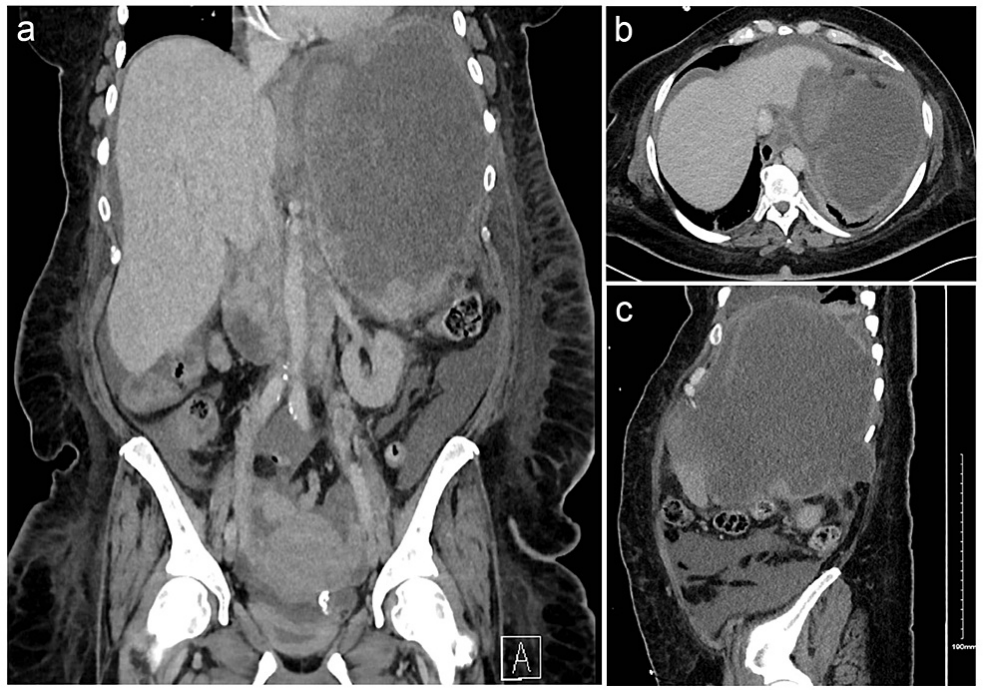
CT abdomen/pelvis with IV contrast, coronal (A), axial (B), and sagittal (C) views on re-admission demonstrating a large heterogeneous cystic septated retroperitoneal mass in the left upper quadrant causing significant mass effect on adjacent organs. The mass is poorly defined anteriorly with increased perisplenic ascites making rupture difficult to exclude. CT: computed tomography; IV: intravenous
